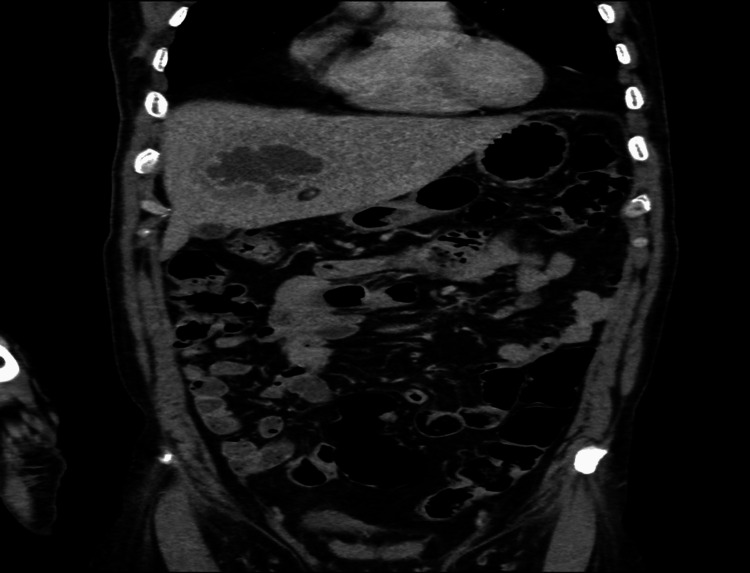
Contrast-enhanced CT imaging showing a well-defined hypodense lesion in the right hepatic lobe with central dense fluid attenuation and peripheral rim enhancement, consistent with hepatic abscesses.
